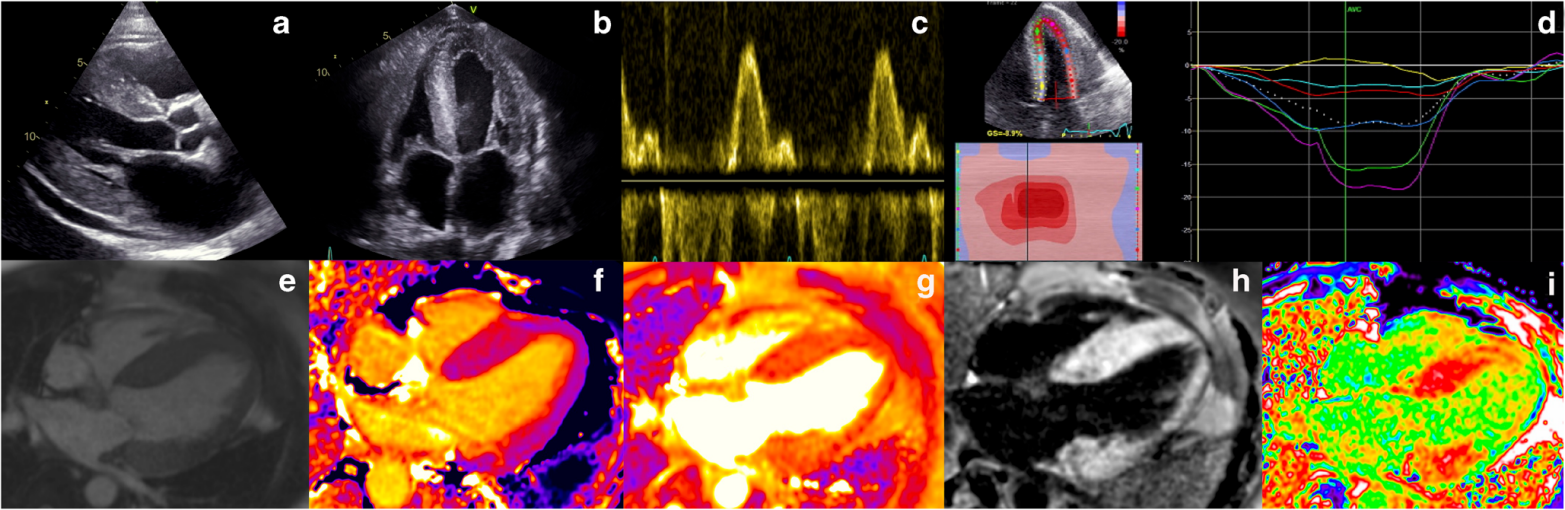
Top Panel: Echocardiography findings in a patient with advanced cardiac amyloidosis. (a) Parasternal long axis view and (b) four chamber view showing concentric left ventricular hypertrophy (c) pulse wave Doppler showing restrictive left ventricular inflow pattern (d) strain pattern characteristic of an infiltrative process. Bottom Panel: CMR findings in a patient with advanced cardiac amyloidosis. (e) Four chamber steady state free precession cine demonstrating left ventricular hypertrophy (f) corresponding native T1 map showing a T1 value of 1150 ms in the basal inferoseptum (g) corresponding T2 map showing a T2 value of 54 ms in the basal inferoseptum, within normal limits (h) corresponding phase sensitive inversion recovery reconstruction showing transmural late gadolinium enhancement (i) corresponding extracellular volume map showing elevated value of 0.70
